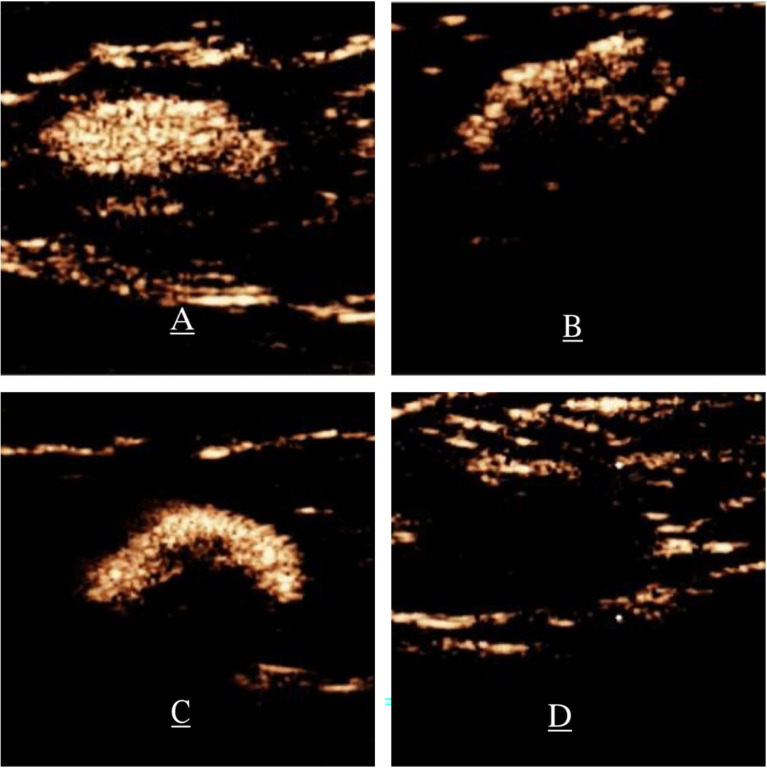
Patterns of enhancement on P-CEUS. (A) Type I, homogenous enhancement. (B) Type II, heterogeneous enhancement, significantly enhanced parenchymal areas of focal hypoperfusion or non-perfusion. (C) Type III, complete or incomplete circumferential ring enhancement and low or no central enhancement. (D) Type IV, slight enhancement or no enhancement. P-CEUS: percutaneous contrast-enhanced ultrasound.
